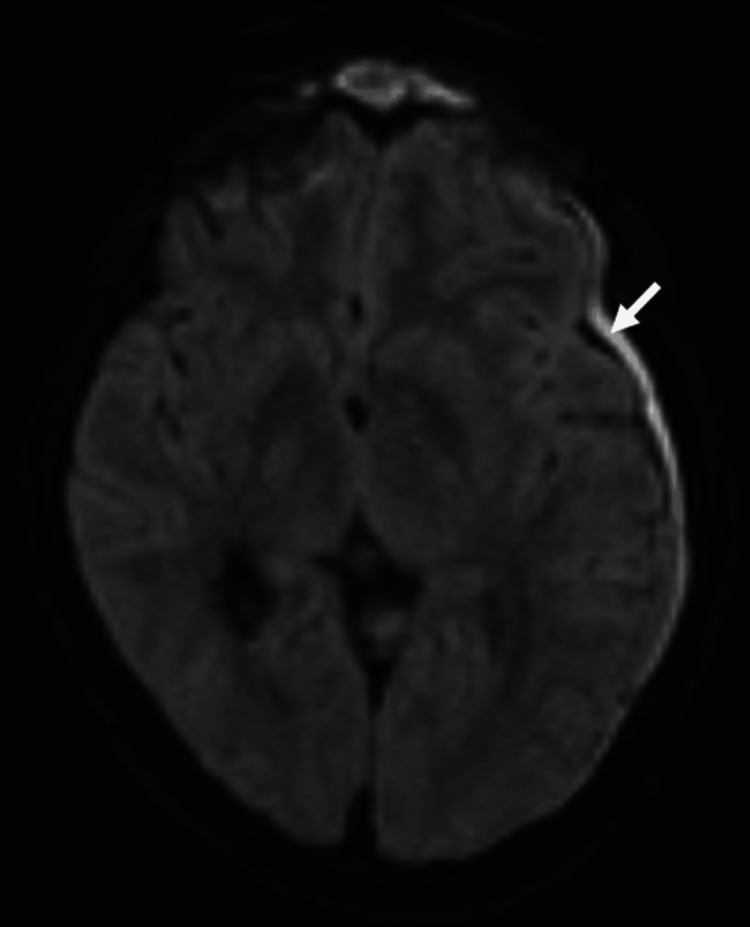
Diffusion-weighted (DWI) magnetic resonance imaging. The arrow indicates the subdural empyema.
